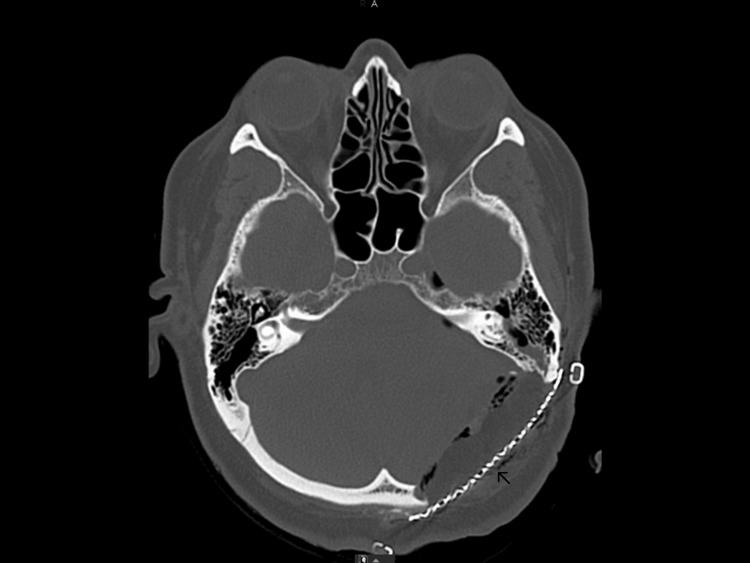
Postoperative CT scan showing reconstruction with a titatinum mesh (arrow).
